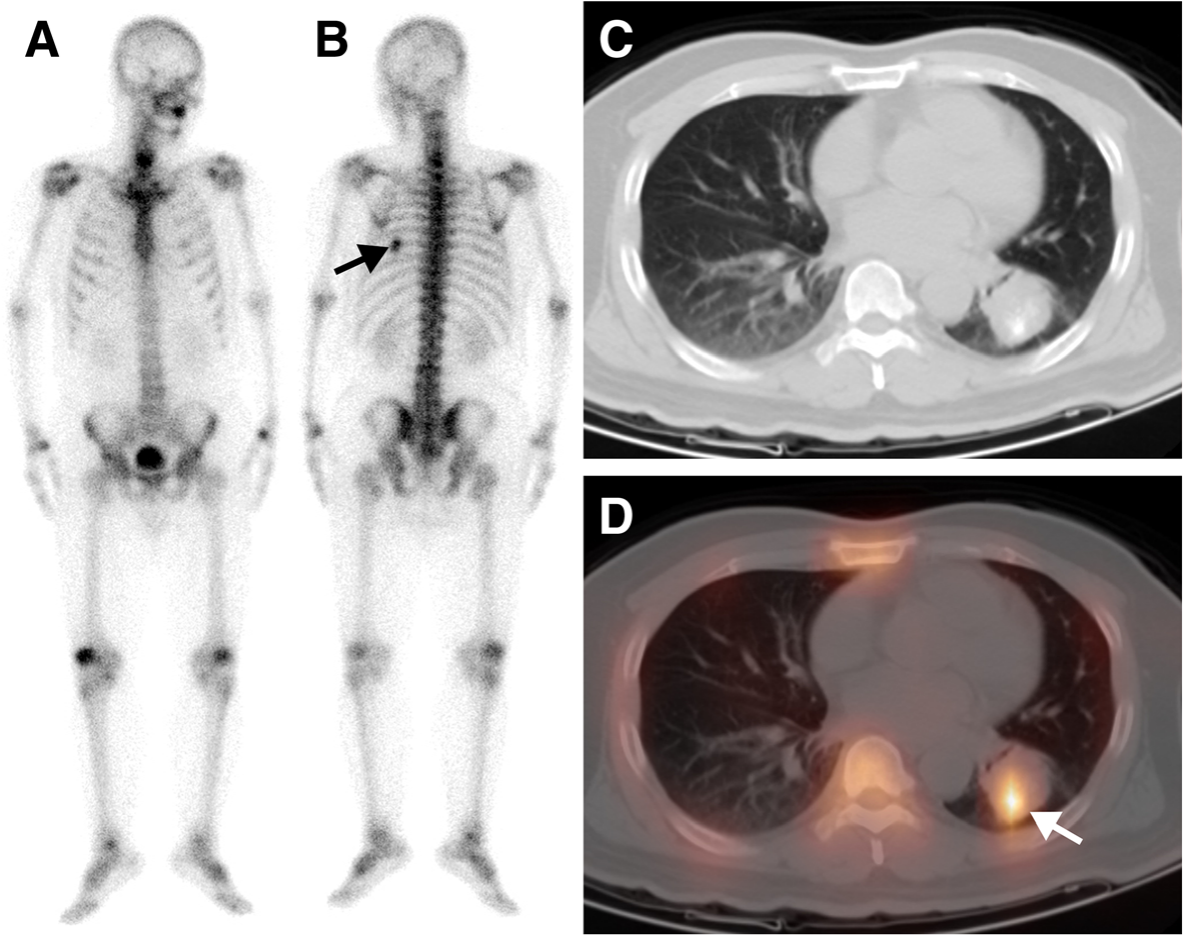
A 66-year-old man with a known gastric stromal tumour was referred for metastatic work-up before surgery. The whole-body scan (a, anterior; b, posterior) revealed a single focus of high ^99m^Tc-MDP activity in the right 7th posterior rib region (black arrow). Axial CT (c), and SPECT/ CT images (d) showed that the elevated ^99m^Tc-MDP activity was located in a pulmonary mass with calcification (white arrow). The patient underwent a left lower lobectomy and pathologic analysis confirmed the diagnosis of a lung adenocarcinoma
